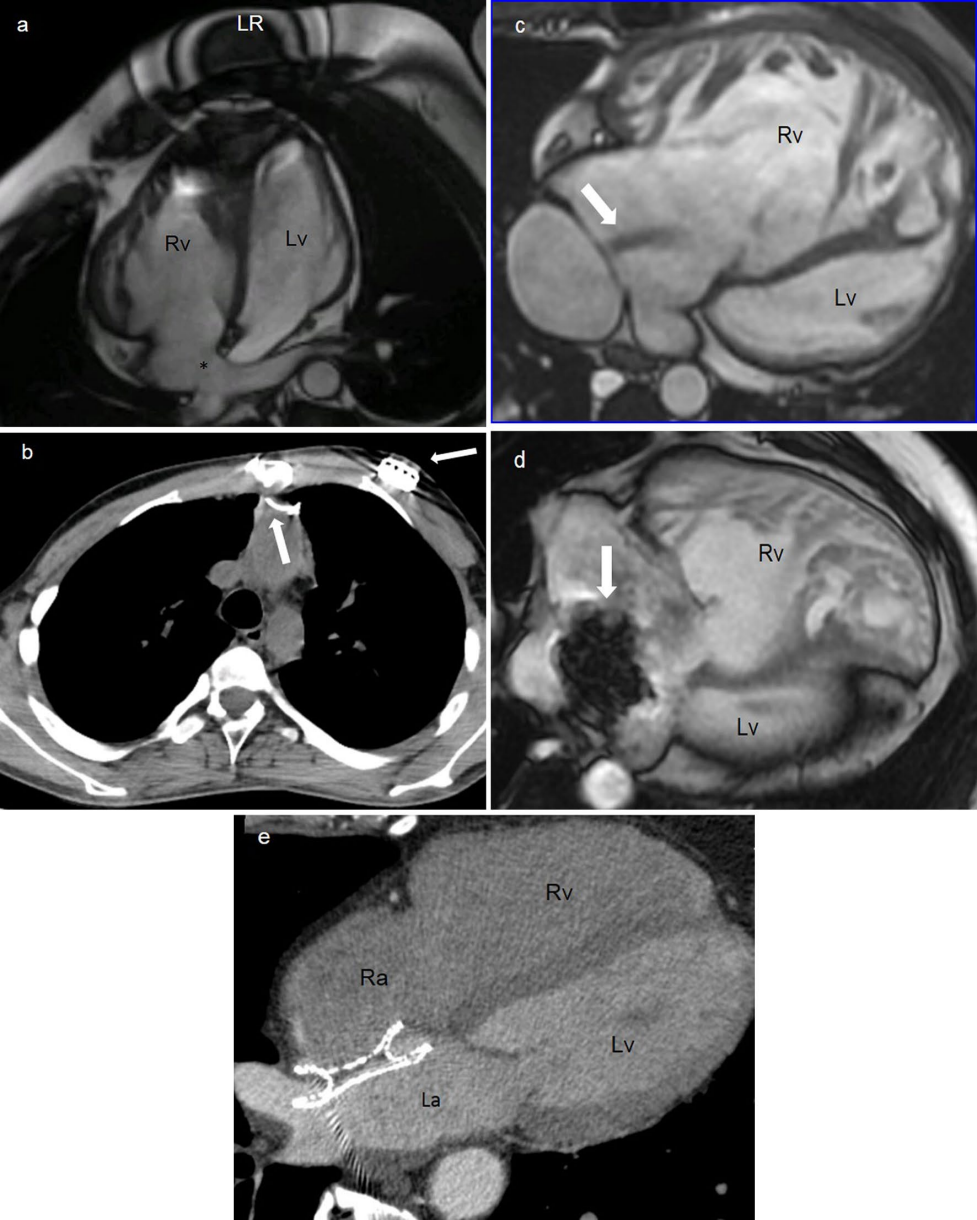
34-year-old male with Mustard repair of c-TGA and loop recorder implantation. CMR SSFP 4-chamber view shows atrio-ventricular concordance and the pulmonary baffle (asterisk). The ventricular apex is canceled by artifacts (a). 18-year-old Fontan patient with pacemaker implantation. CCT axial plane displays the wires and the pacemaker generator (arrows) with minimal artifacts upon thoracic aorta (b). Adult male patient with Mustard repair of c-TGA and baffle leakage. CMR SSFP 4-chamber images show the flow turbulence (arrow) before the treatment (c) and a huge artifact (arrow) caused by the metallic closure device (d). Adult male with ASD after endovascular closure. CCT axial image well depicts the closure device without limitations to cardiac chambers visualization (e). *CCT* cardiovascular computed tomography, *CMR* cardiovascular magnetic resonance, *cTGA* complete transposition of the great arteries, *SSFP* Steady State Free Precession, *La* left atrium, *Lv* left ventricle, *Ra* right atrium, *Rv* right ventricle
